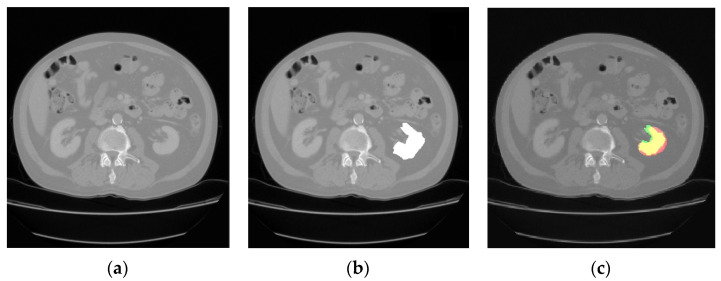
CT image of a healthy kidney (a); the medical expert segmentation (shown in white) (b); and the result of the model’s prediction (c).
